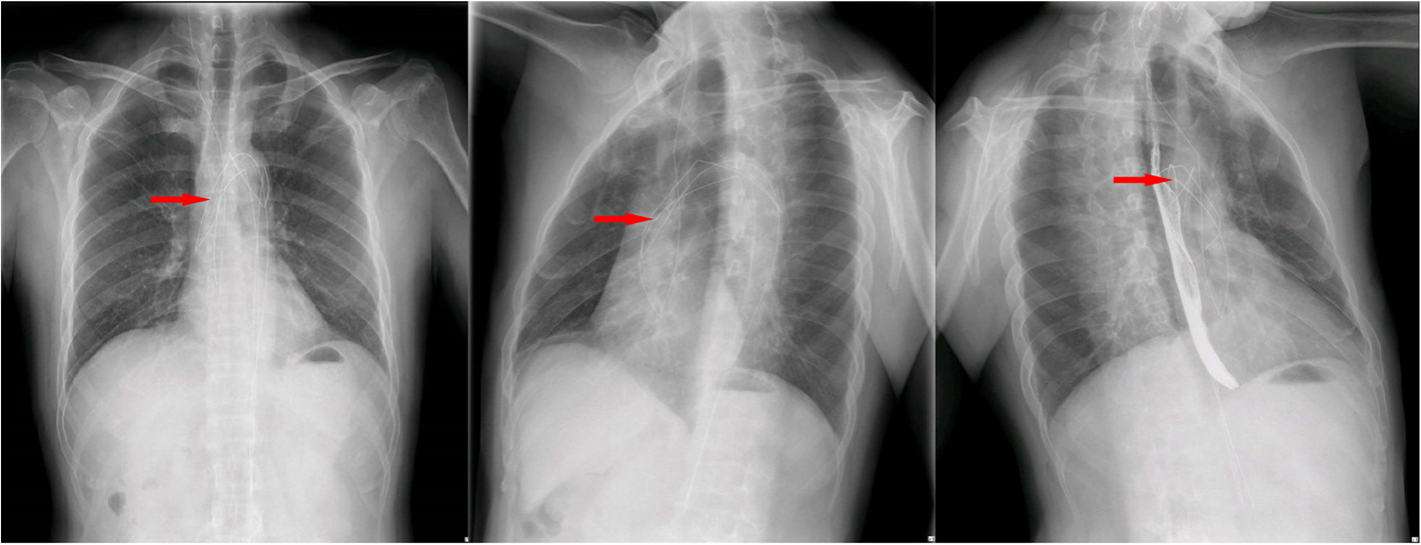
Cardiac three-dimension radiograph showed a linear high-density shadow in the aorta, which partly wrapped around the aortic arch, and one end entered the right common carotid artery (red arrow)
